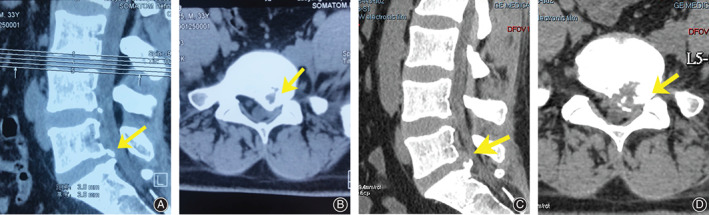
A 33‐year‐old male patient was hospitalized for pain and numbness of the left lower extremity for 6 months. (A & B) Preoperative CT images; his diagnosis was L_5_S_1_ posterior ring apophyseal fracture. (C & D) Postoperative CT images. After percutaneous transforaminal endoscopic discectomy, most of the bones were removed, and a small amount of bone remained. Soft tissue was removed and symptoms of compression were relieved.
